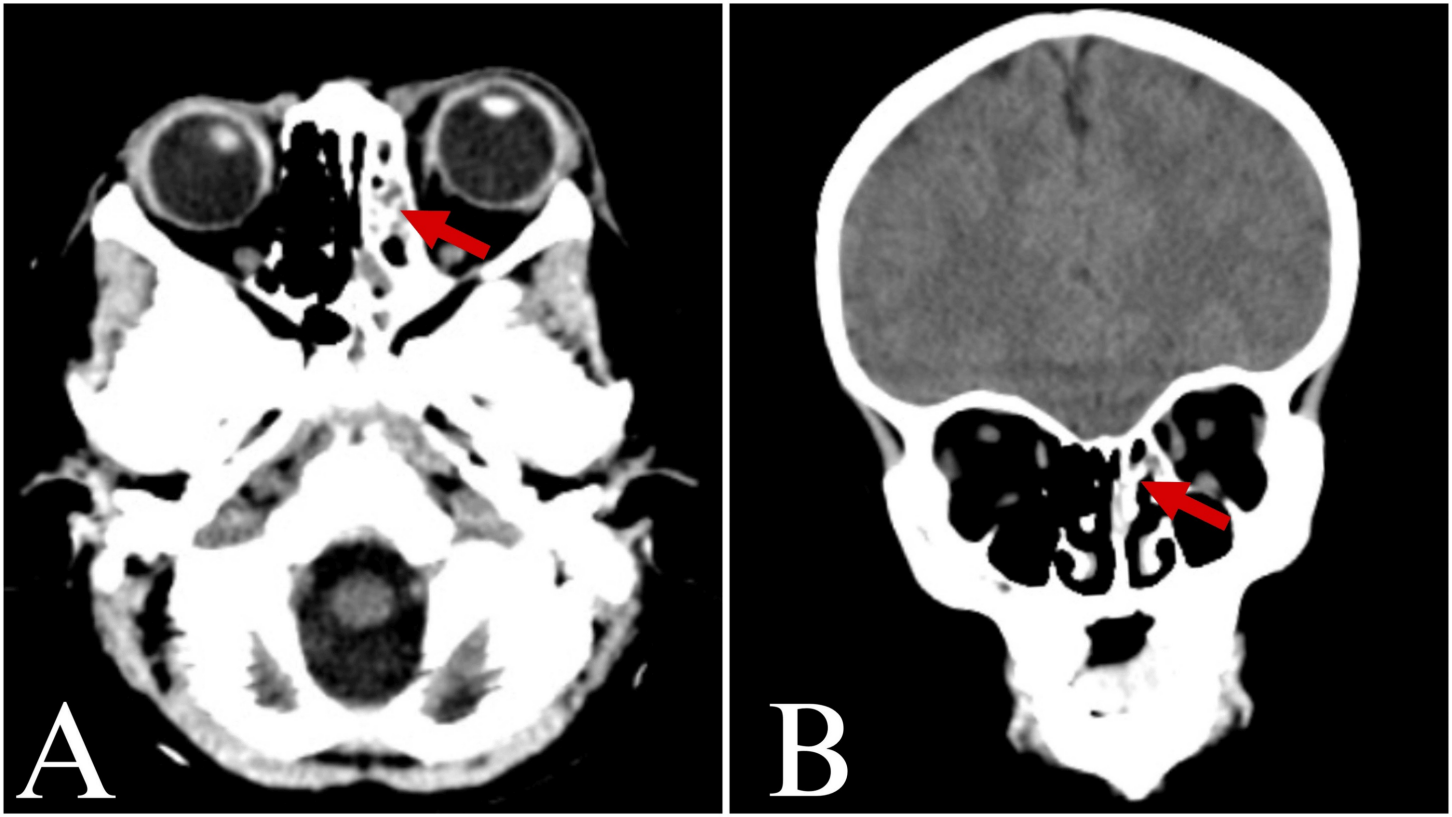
CT imaging demonstrated minimal orbital emphysema with associated left periorbital soft tissue edema (red arrow) (A) Coronal and (B) axial views of the head and orbits
